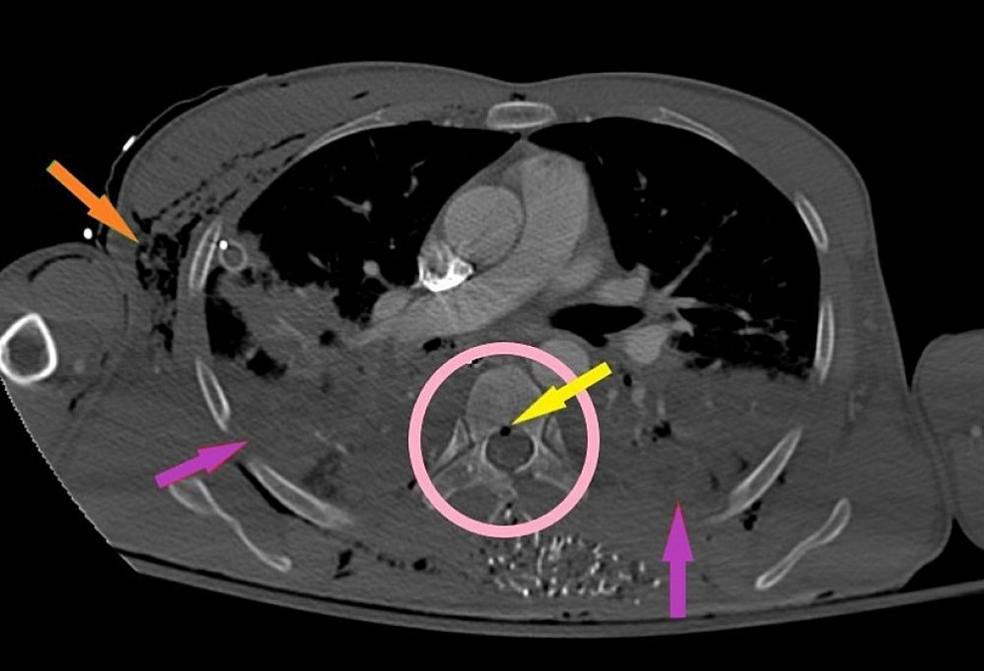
Contrast-enhanced CT with an axial bone window of the chest. The orange arrow points to subcutaneous emphysema. The purple arrow points to bilateral hemorrhagic pleural effusions. The pink circle highlights the right costovertebral junction, right pedicle, and left lamina of T6 that is completely shattered. The yellow arrow points to hemorrhagic material in the spinal cord with punctate foci of gas. CT: computed tomography
